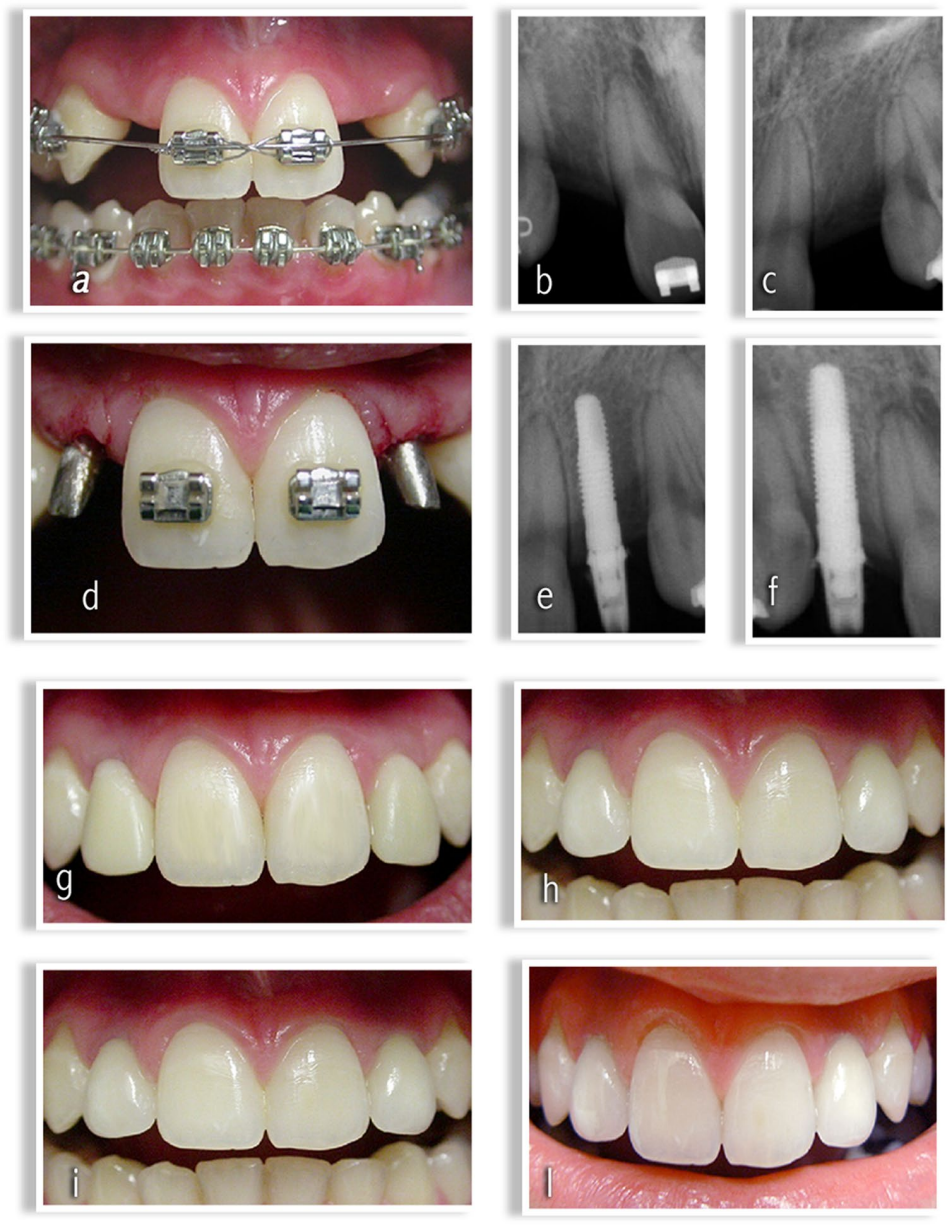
a Maxillary lateral incisors agenesis: orthodontic opening of the space in regions 1.2 and 2.2. b Radiographic evaluation of alveolar crest in region 1.2. c Radiographic evaluation of alveolar crest in region 2.2. d Post-surgical implant sites in regions 1.2 and 2.2. e Radiographic evaluation post-surgical implant site in region 1.2. f Radiographic evaluation post-surgical implant site in region 2.2. g Peri-implant tissue aspect after 1 month. h Peri-implant tissue aspect after 1 year. i Peri-implant tissue aspect after 5 years. l Peri-implant tissue aspect after 10 years.
